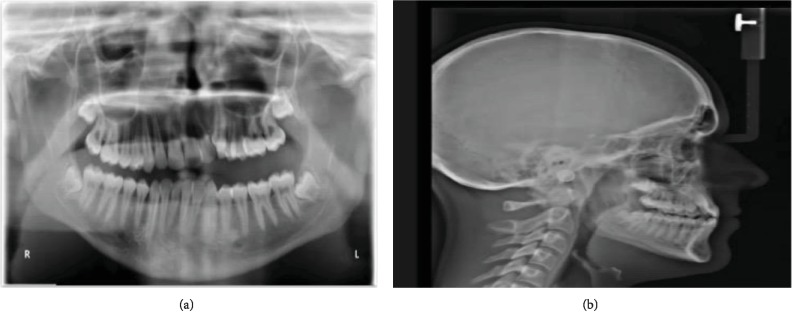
(a) Pretreatment panoramic X-ray showing all teeth present without any pathology and unlevelled upper and lower left posterior segments. (b) Pretreatment cephalometric X-ray of the patient's skeletal class I pattern with an increase in the length of the mandible and maxilla and increased lower facial height.
